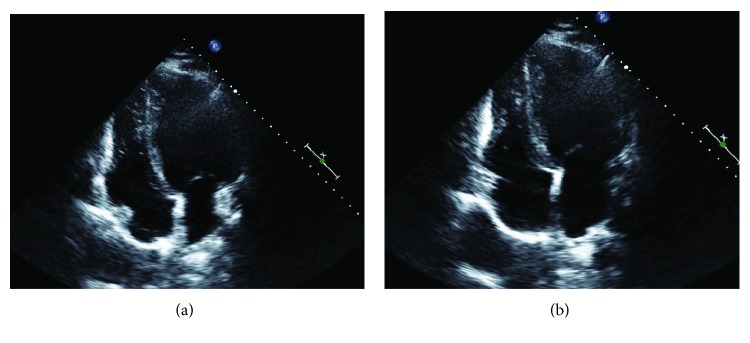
Echocardiogram on the day of presentation in (a) diastole and (b) systole (bar scale: one integer = 1 cm).
